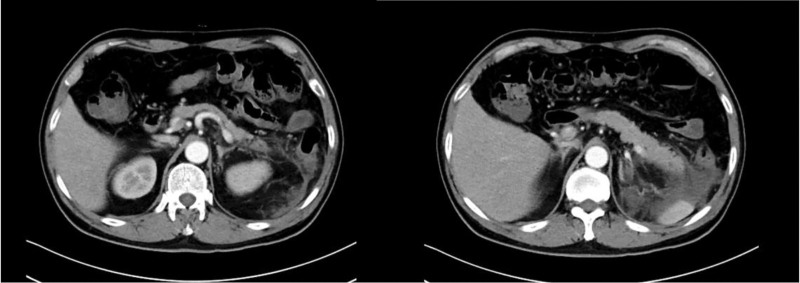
Whole abdomen enhanced CT showed exudation and effusion of pancreatic body and tail. CT = computed tomography.
